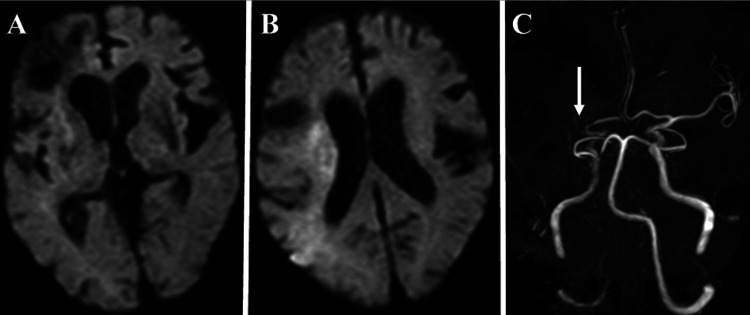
Head magnetic resonance imaging (MRI) at presentation. (A, B) MRI diffusion-weighted images. High-intensity signals are seen in the right putamen, corona radiata, and parieto-temporal lobes. (C) Magnetic resonance angiography (MRA) showing poor visualization beyond the origin of the right middle cerebral artery (white arrow).
